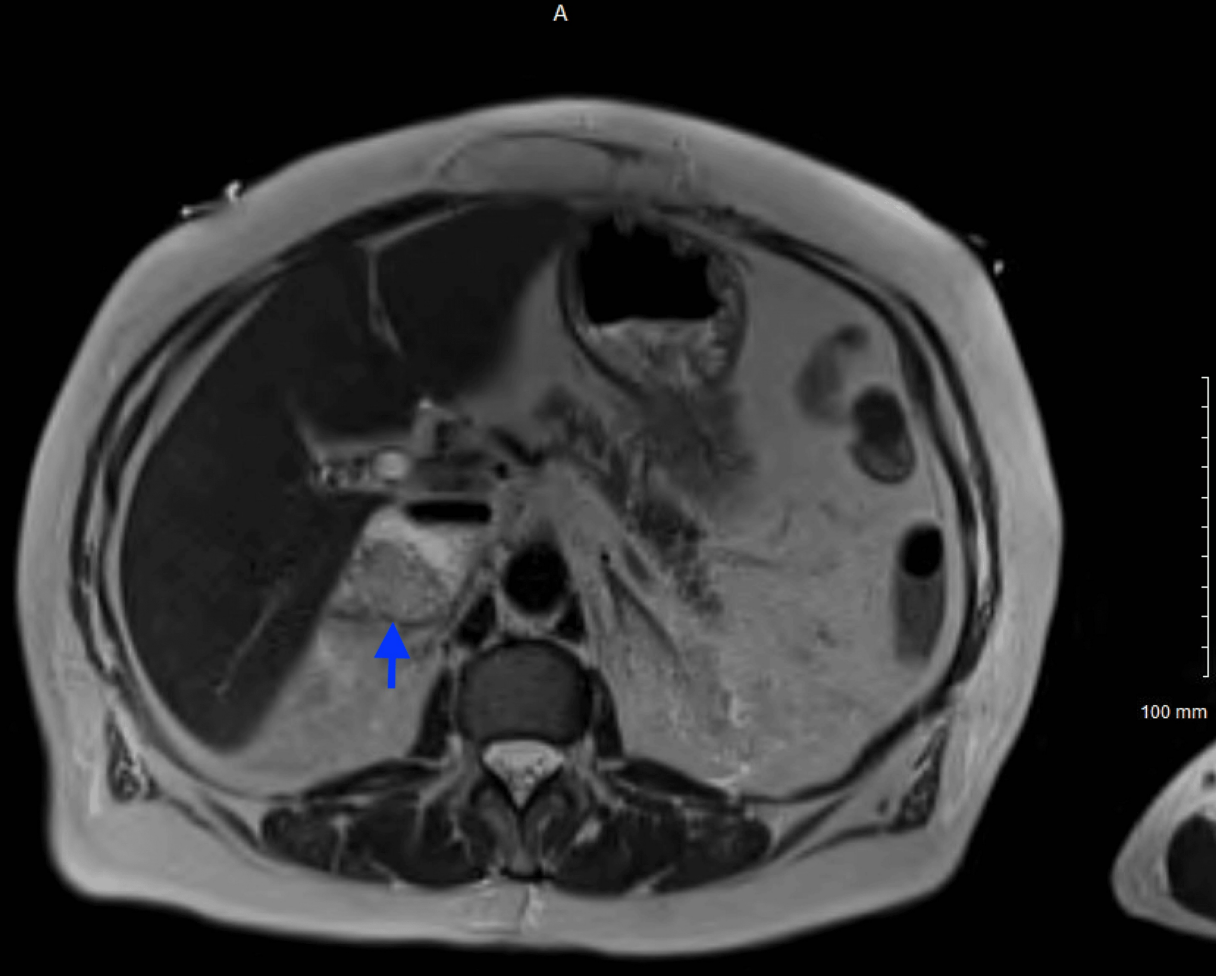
MRI abdomen without contrast T2 HASTE TRA-P2 series (axial view) MRI abdomen showing a lobulated, 5.8 x 4.6 x 3.8 cm heterogeneous mass (blue arrow) of the right adrenal gland.
